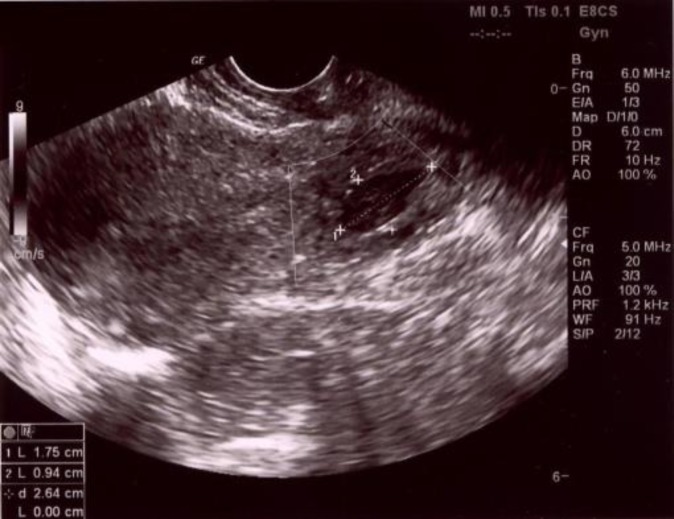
Ultrasonic picture of cervical pregnancy
